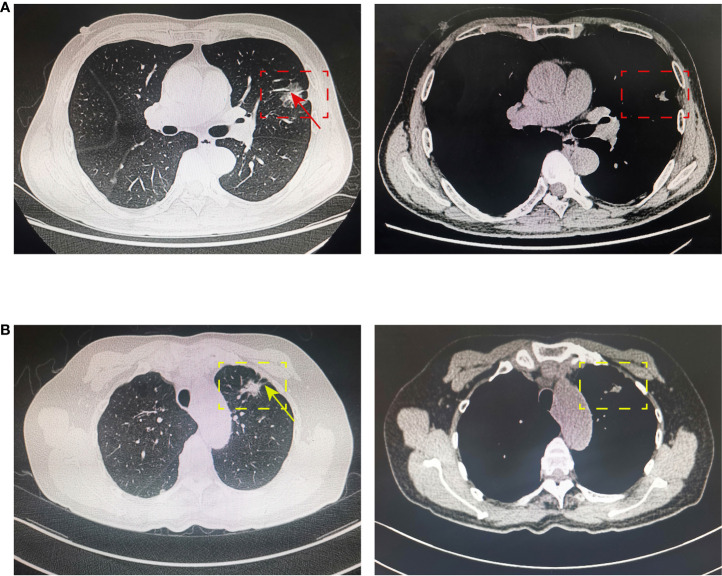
Bronchial cutoff and spiculation sign on CT. (A) The bronchial cutoff sign of PSILA is shown in the pulmonary window (left) and mediastinal window (right). The red box shows the location of the tumor, and the red arrow shows the classical bronchial cutoff sign. (B) The spiculation sign of PSILA is shown in the pulmonary window (left) and mediastinal window (right). The yellow box shows the location of the tumor, and the yellow arrow shows the classical spiculation sign. .
